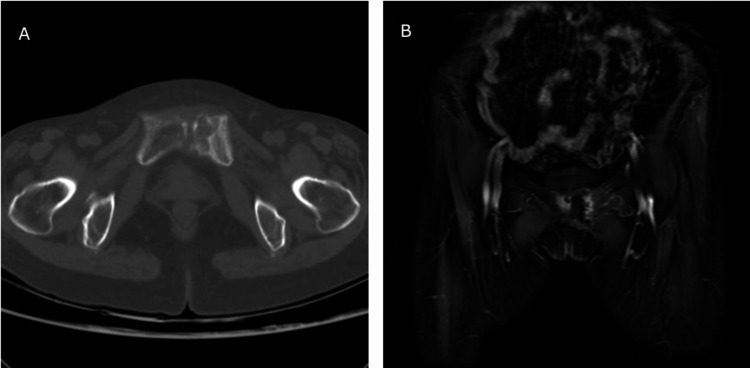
Imaging examination of the pubic bone (A) CT scan showing destruction and fracture of the left superior pubic ramus. (B) MRI showing hypointense on T1-weighted images, isointense, and hyperintense on T2-weighted images.
